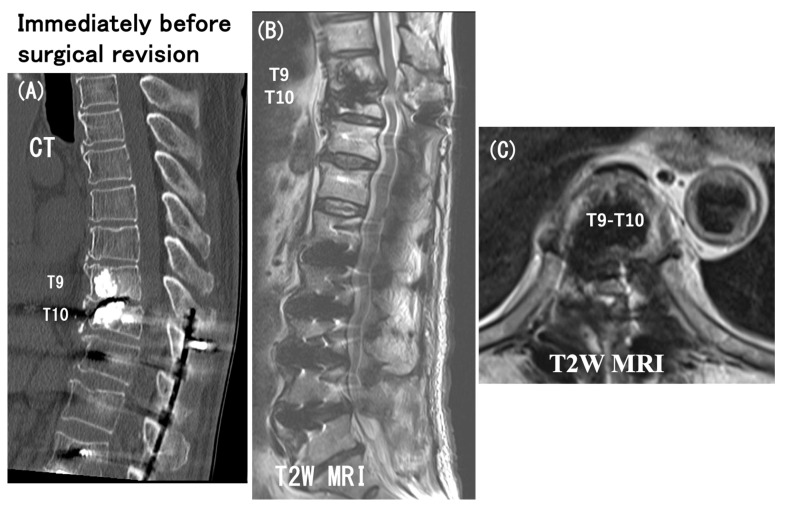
Sagittal reformatted CT scan (A), sagittal T2 weighted MRI (B), and axial T2 weighted MRI at T9-T10 level (C) obtained immediately before surgical revision. These images more clearly depict the UIV fracture without the screw pullout and distinct spinal cord compression at the T9-T10 intervertebral level.
